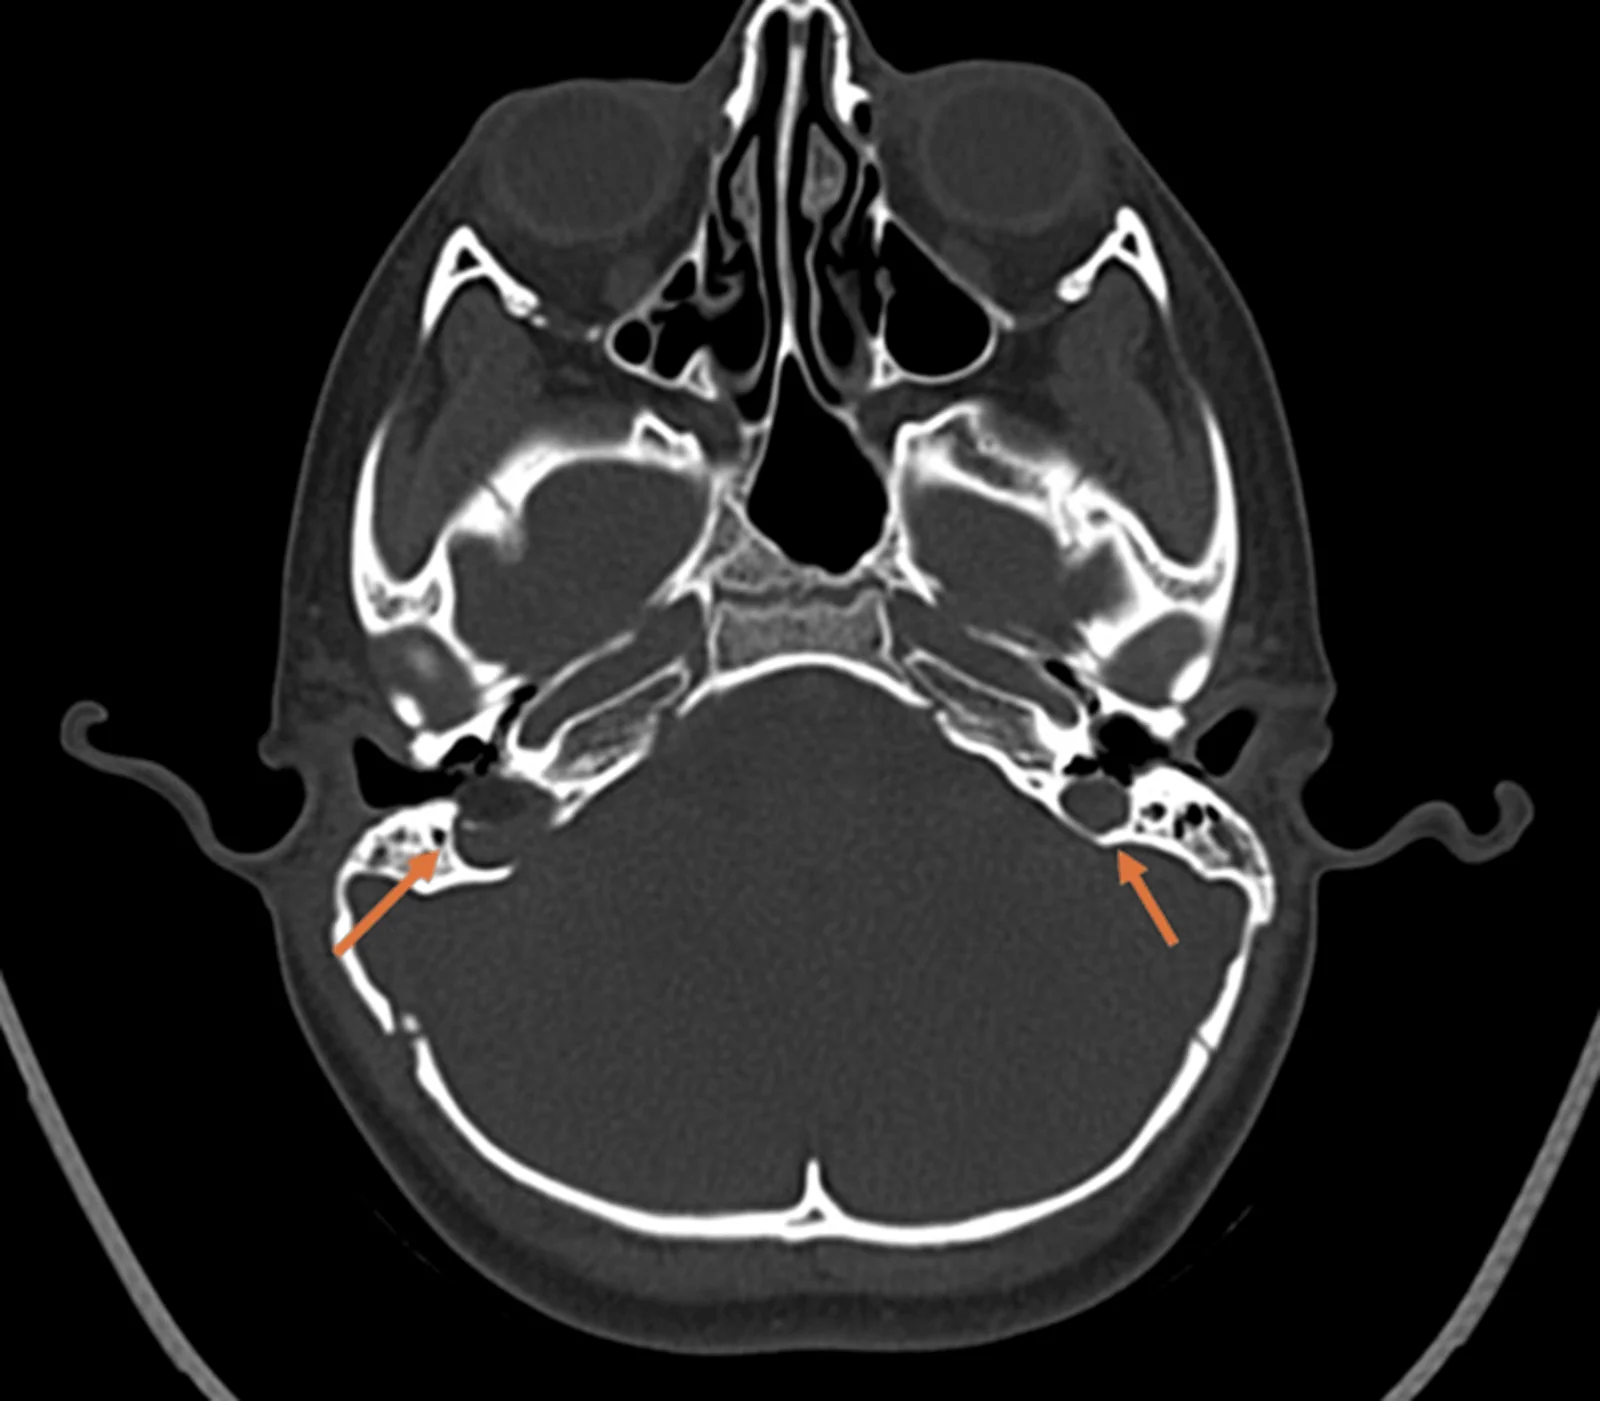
Axial section of CT scan of the temporal bones without contrast, revealing bilateral DJB covered by thin bony plate. (arrows on both sides are indicating the DJB). DJB: Dehiscent jugular bulb.
